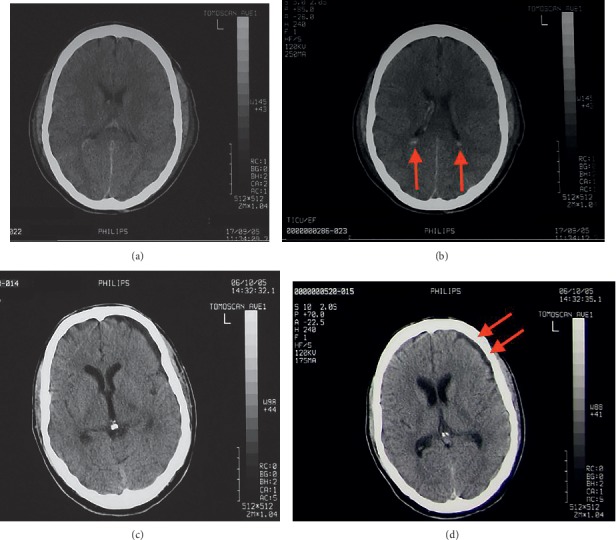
CT scans of the patient brain immediately after (a and b) and 19 days after accident (c and d). The images (a) and (b) above show some intraventricular hemorrhage (arrows show intraventricular hemorrhage in the posterior horns of the lateral ventricles) after accident. In the images (c) and (d), the intraventricular hemorrhage has resolved completely. Arrows show suspected new discrete atrophic changes in the left frontal lobe with sulcal prominence that was not present in the initial scans.
